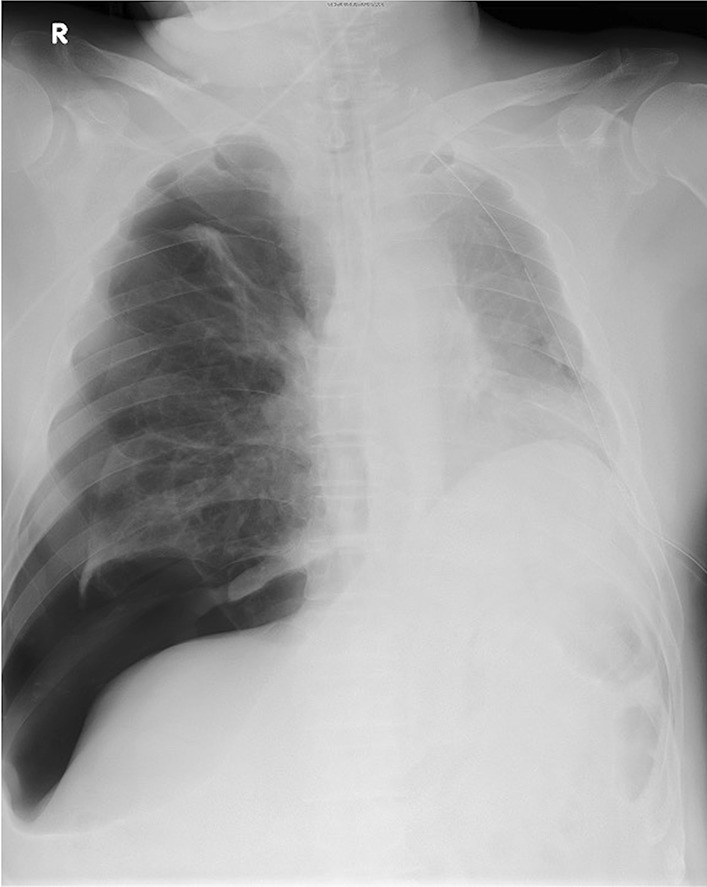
Chest x-ray using a portable s-ray system reveals a right-sided tension pneumothorax after the surgery
